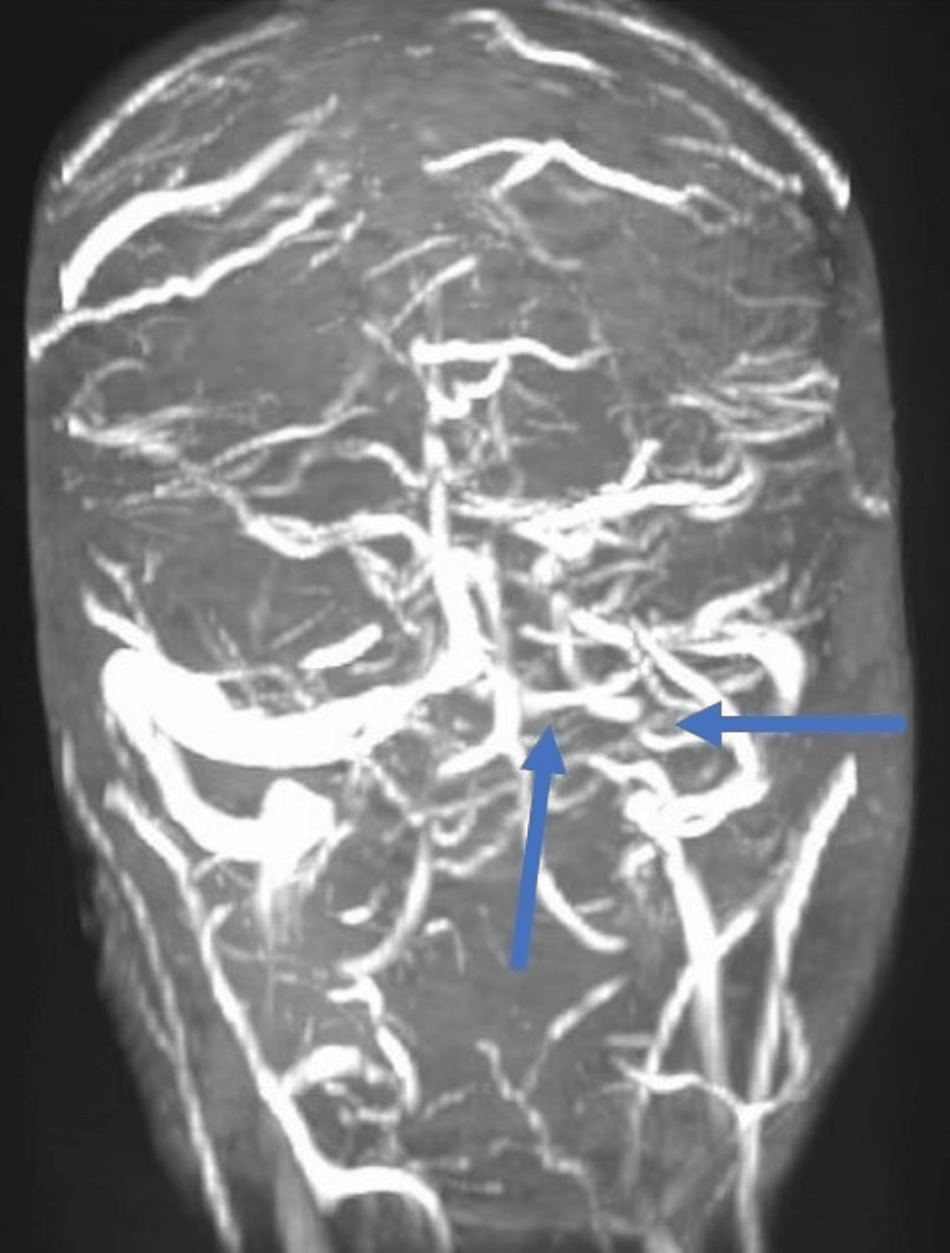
CT venography showing a filling defect in the left transverse sinus (arrows showing the absence of the left transverse sinus)
